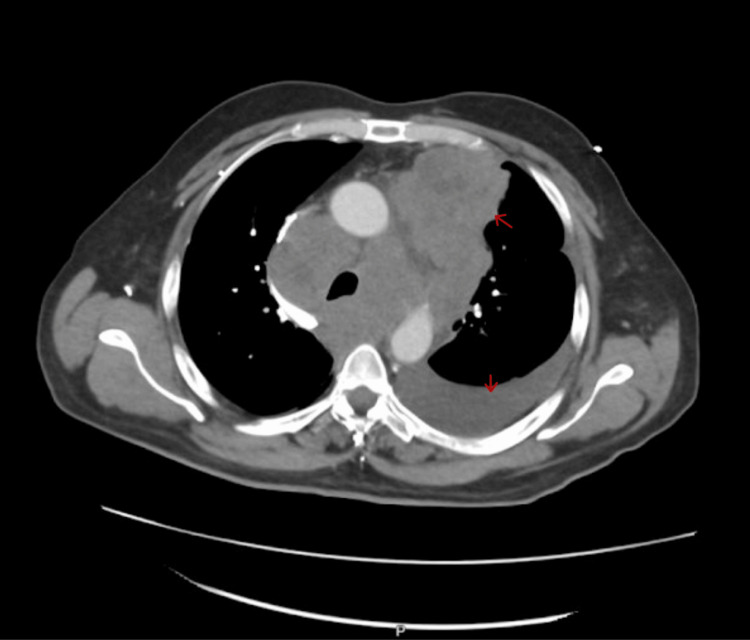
CT chest with contrast axial view shows mediastinal mass with left-sided pleural effusion and extensive mediastinal adenopathy
